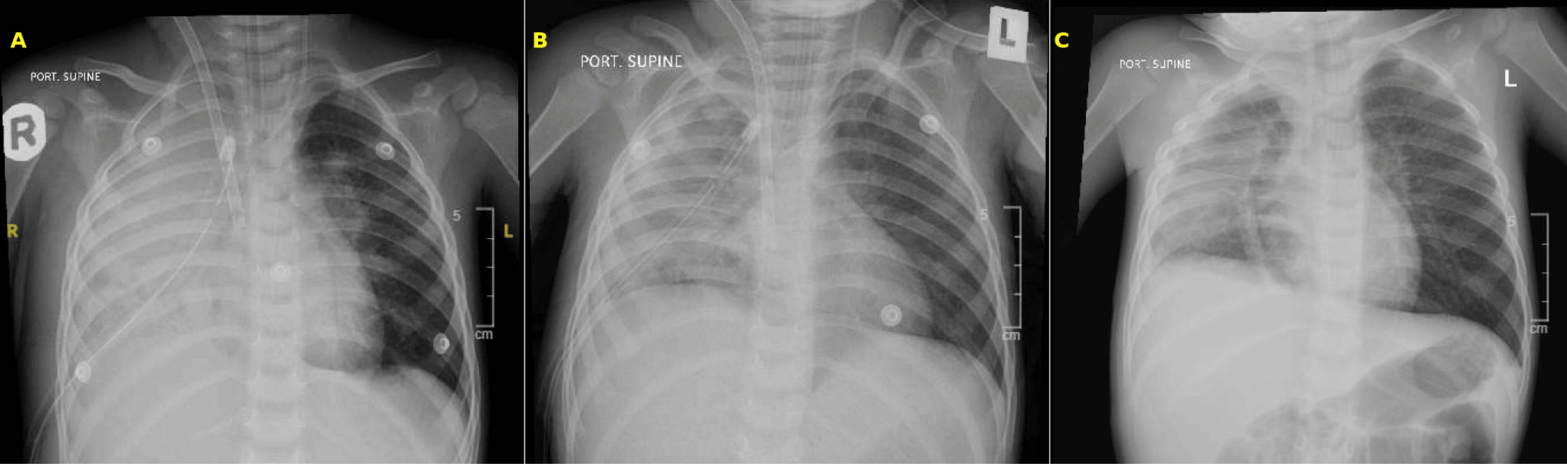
Portable supine anteroposterior chest radiographs from Case 1. (A) Day 0, while on venovenous extracorporeal membrane oxygenation (VV-ECMO): yellow “A” label highlights dense confluent consolidation with small internal lucencies occupying the right middle and lower lobes - early cavitation typical of pediatric necrotizing pneumonia. (B) Day 4 on VV-ECMO: yellow “B” label indicates a pleural drain coursing inferiorly within the now near-homogeneous right-sided opacity and volume-loss pattern, representing postoperative atelectasis and residual pleural fluid. The mediastinum remains mid-line. (C) Day 14 (48 h after ECMO decannulation): yellow “C” label points to coarse linear parenchymal scarring and sub-segmental atelectasis after marked re-aeration of the right lung, indicating resolution of the necrotizing process.
